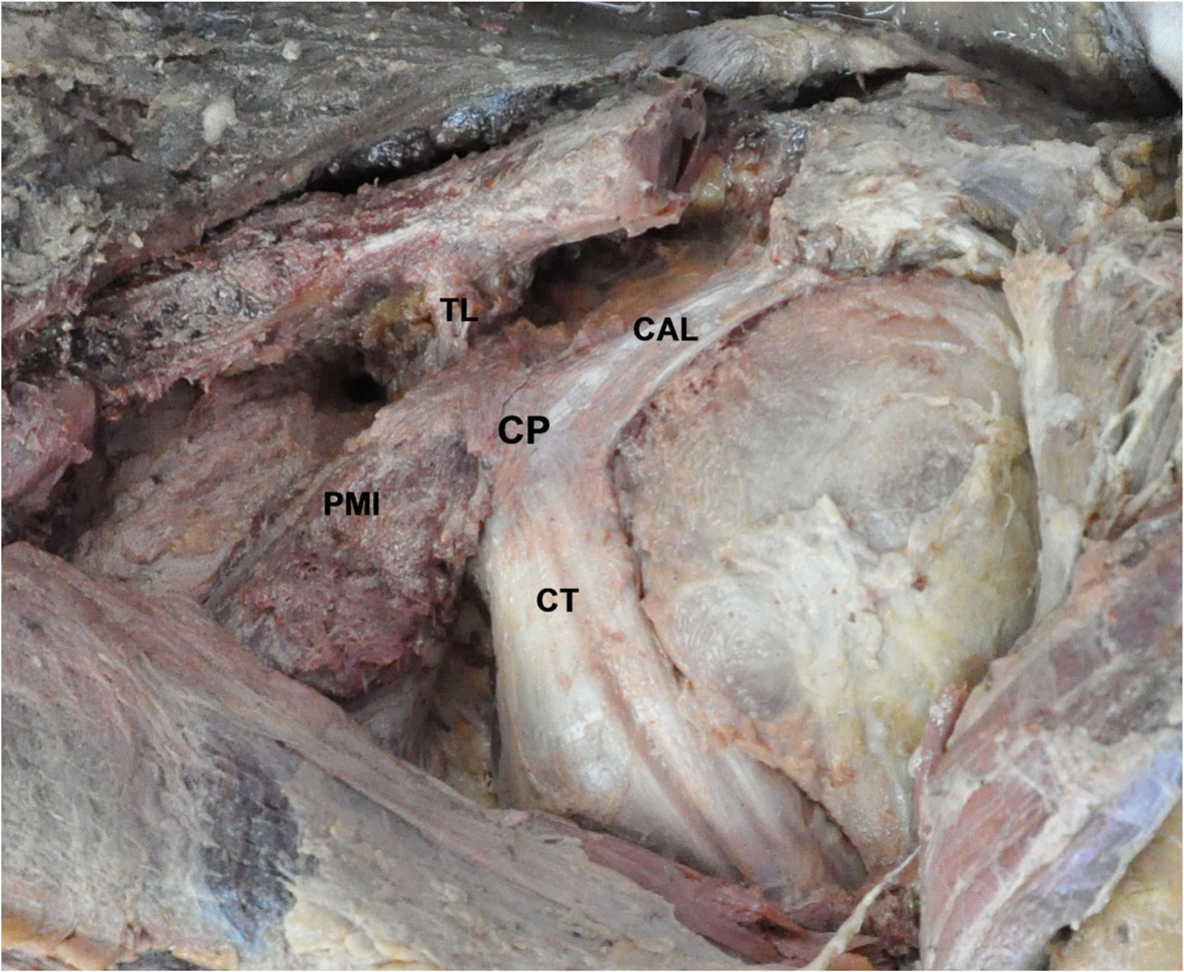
Anatomical structures in an isolated coracoid process of the left shoulder. *CAL* coracoacromial ligament, *CP* coracoid process, *CT* conjoint tendon, *PMI* pectoralis minor, *TL* trapezoid ligament
